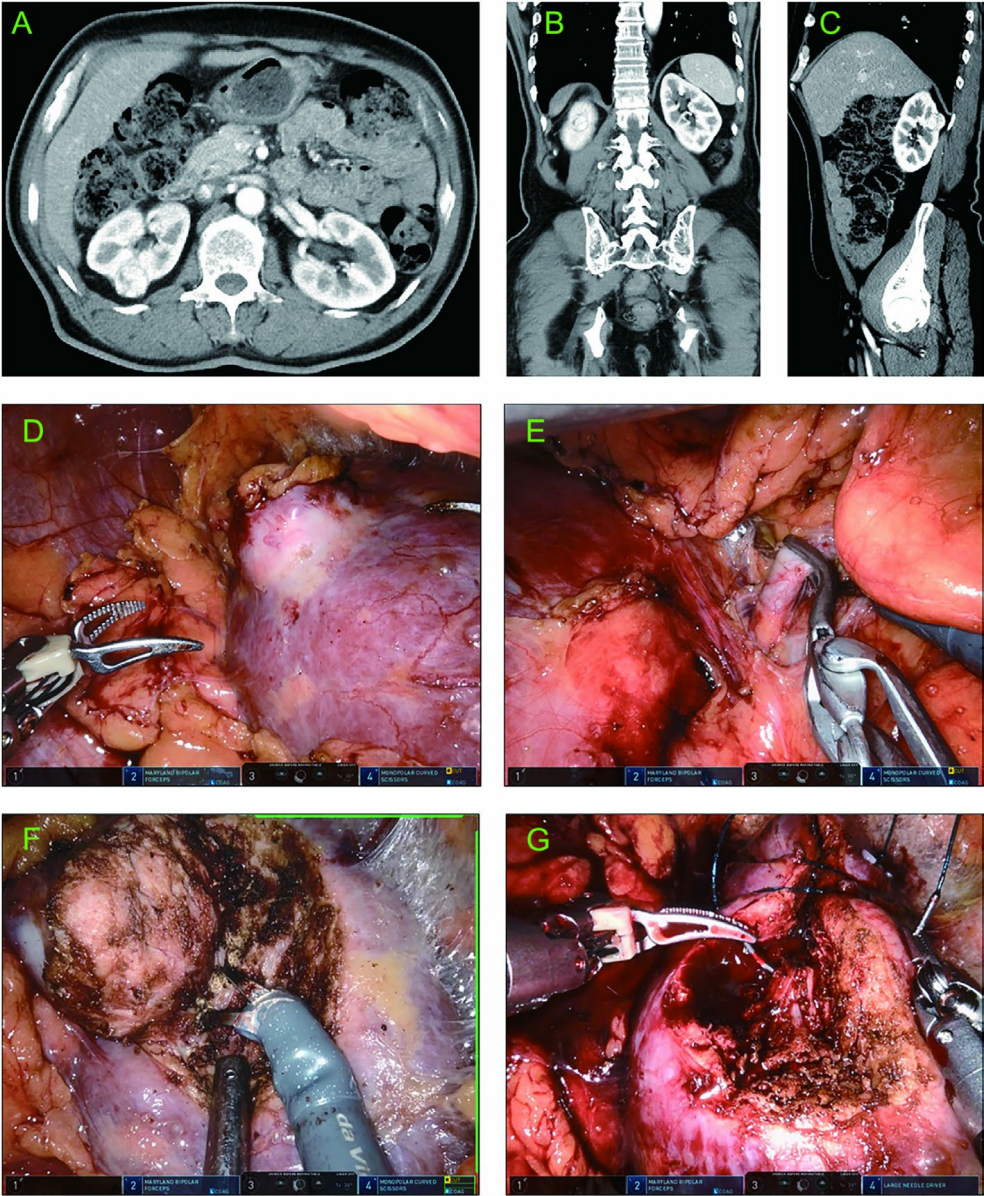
A typical case of the retroperitoneal approach. (A–C) Contrast‐enhanced CT demonstrated a rounded and enhanced‐density mass measuring approximately 2.3 cm; (A), (B), and (C) showed the horizontal, coronal, and sagittal images, respectively. (D) The tumor was well exposed in the retroperitoneal space with a clear field of view. (E) The renal artery was clamped. (F) The tumor was removed using a robotic arm without involvement of the collecting system. (G) The wound after tumor resection was sutured.
